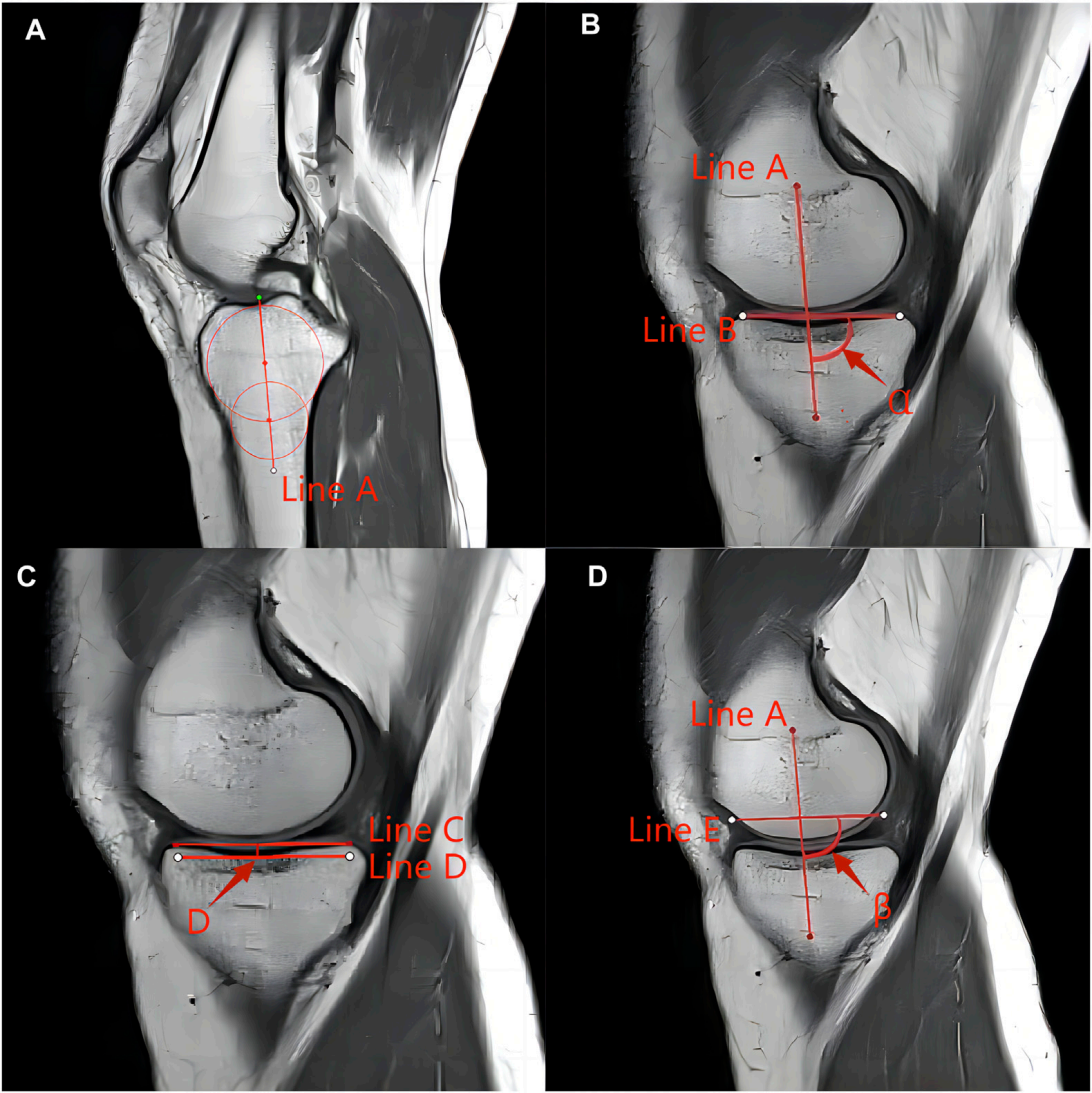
Measurement techniques of determining the posterior tibial slope in the MRI according to the method described by Hudek et al. (A) Determination of the proximal anatomical axis of the tibia. Localization of the central sagittal plane is performed in the sagittal position. The plane includes the posterior cruciate ligament termination point, the intercondylar eminence, and the anterior and posterior tibial cortical concave. Two circles are drawn on the proximal tibia, the proximal circle is tangent to the anterior and posterior tibial cortex and tibial plateau, and the distal circle is tangent to the anterior and posterior tibial cortex, and the center of the circle is on the proximal circle center. The center of the circle connecting the two circles is the anatomical axis of the proximal tibia (line A). (B) Measurement of the posterior slope angle of the tibial plateau: determine the middle sagittal plane of the medial tibial plateau (the midpoint from the intercondylar spine to the edge of the medial tibia), connect the anterior and posterior margins of the uppermost tibia with the cortex, and draw a tangent line to the medial plateau (line B). Duplicate the proximal tibial anatomical axis in this plane (line A), and measure the angle between the vertical line of the proximal tibial anatomical axis and the medial tibial plateau tangent as the medial tibial plateau posterior tilt angle (90°-α). Similarly, the posterior inclination of the lateral tibial plateau was measured in the same way. (C) Depth of the medial tibial plateau: in the middle sagittal plane of the medial tibial plateau, connecting the uppermost anterior and posterior margins of the tibial plateau with the cortex, draw a tangent line of the medial plateau (Line C), and then at the lowest point of the concave surface of the tibial plateau draw a tangent line parallel to this line (Line D), which represents the lowest boundary of the subchondral bone, and measure the perpendicular distance between the 2 lines as the depth of the medial tibial plateau (“D” in Figure 3c). (D) Measurement of posterior slope of the meniscus: in the sagittal plane in the middle of the medial tibial plateau mentioned above, connect the anterior and posterior corners of the meniscus (Line E), copy the anatomical axis of the proximal tibia in this plane, and measure the angle between the plumb line of the proximal tibial anatomical axis and the tangent line of the meniscus, i.e., the posterior inclination of the medial meniscus (90°-β). Similarly, the posterior inclination of the lateral meniscus was measured in the same way.
